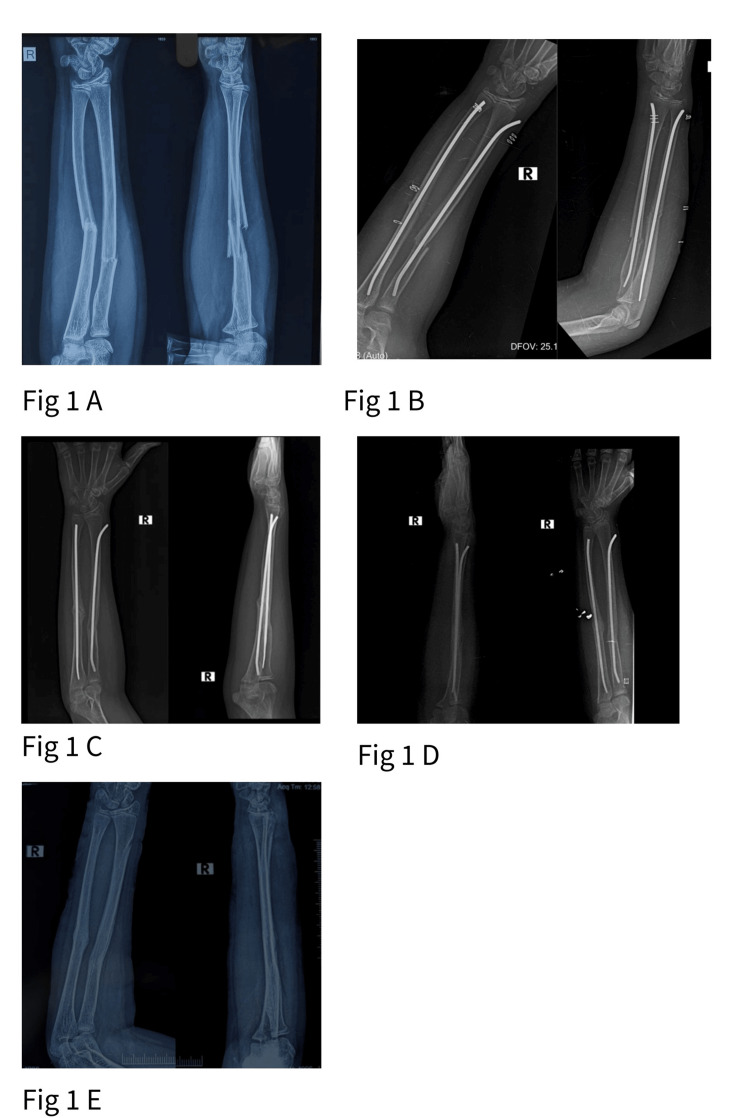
Plain radiographs representing retrograde titanium elastic nailing for right ulna fracture A: Pre-operative; B: Immediate post-operative; C: 6 months follow-up; D: 9 months follow-up with complete union; E: post-implant removal.
